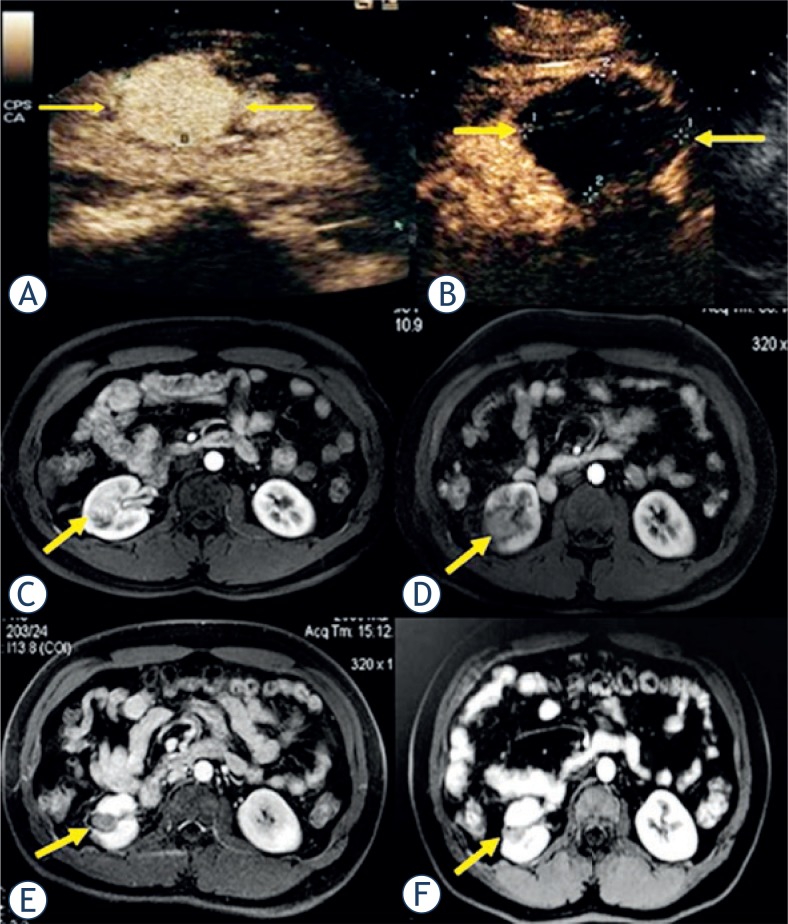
Pre-ablation contrast-enhanced US showed a hyper-enhancement 2.4 cm × 1.9 cm renal clear cell carcinoma in renal parenchyma in a 45-year-old man (A, arrow). Transverse contrast-enhanced MR imaging showed one hyper-intense lesion adjacent to renal pelvis pre-ablation (C) and hypo-intense in arterial phase 7 days (D), 1 year (E) and 2 years (F) after ablation, and the lesion shrunk gradually obviously.
